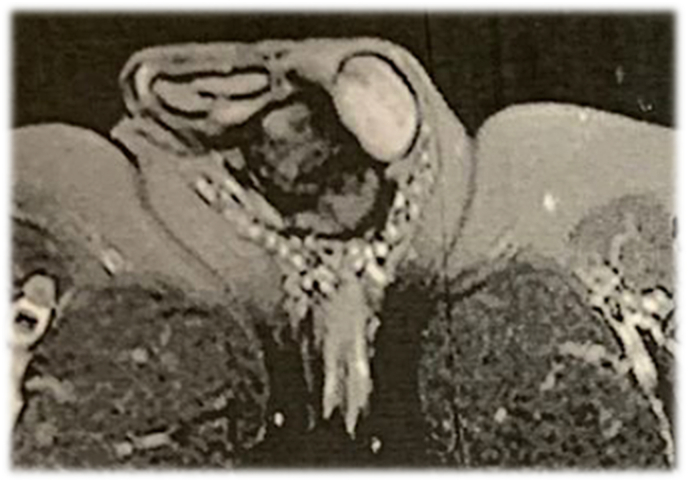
Pre-operation MRI that shows a low T1 high T2 subcutaneous lesion measuring 27*21 mm in left side of penis.
